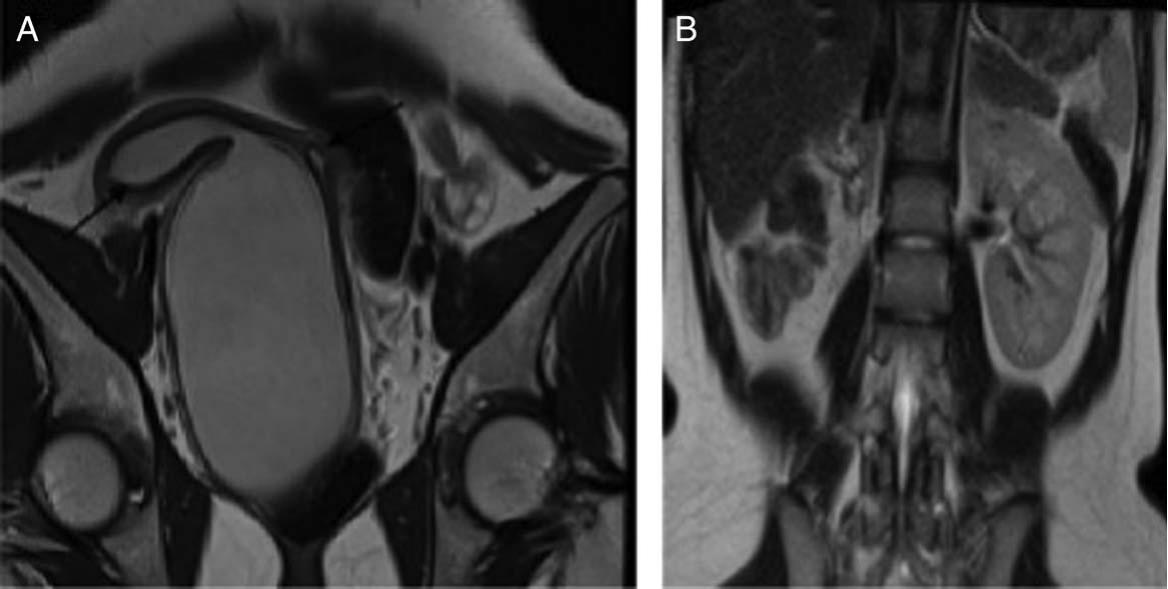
A 14-year-old female with dysmenorrhea. (A) Coronal T2-weighted image shows a didelphic uterus (black arrows) with high signal content in the right cervix and vagina. Mild distension of the right uterine cavity, left uterine, and cervical canal is collapsed. (B) Agenesis of the right kidney is shown.
